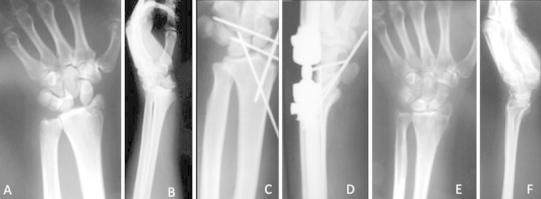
Posteroanterior (a) and lateral (b) radiographs of a 33-years-old female showing a trans-styloid perilunate fracture dislocation. Postoperative posteroanterior (c) and lateral (d) views of the same wrist after open reduction and application of external fixator and additional use of K-wires. Posteroanterior (e) and lateral (f) radiographs 12 months postoperatively showing normal carpal alignment with no signs of arthritis
